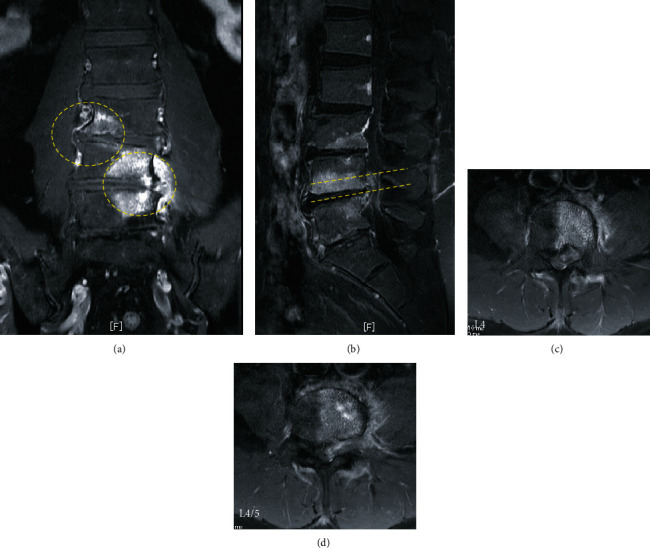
Contrast-enhanced MRI scans in coronal (a), sagittal (b), and axial (c, d) views reveal an epidural mass at L4/5 and bone defects with concentric rim of edema (circle) (hypointense in T1-weighted, hyperintense in T2-weighted and short tau inversion recovery (STIR)) in the endplates of the L3, L4, and L5 vertebrae and acute Schmorl's nodes.
